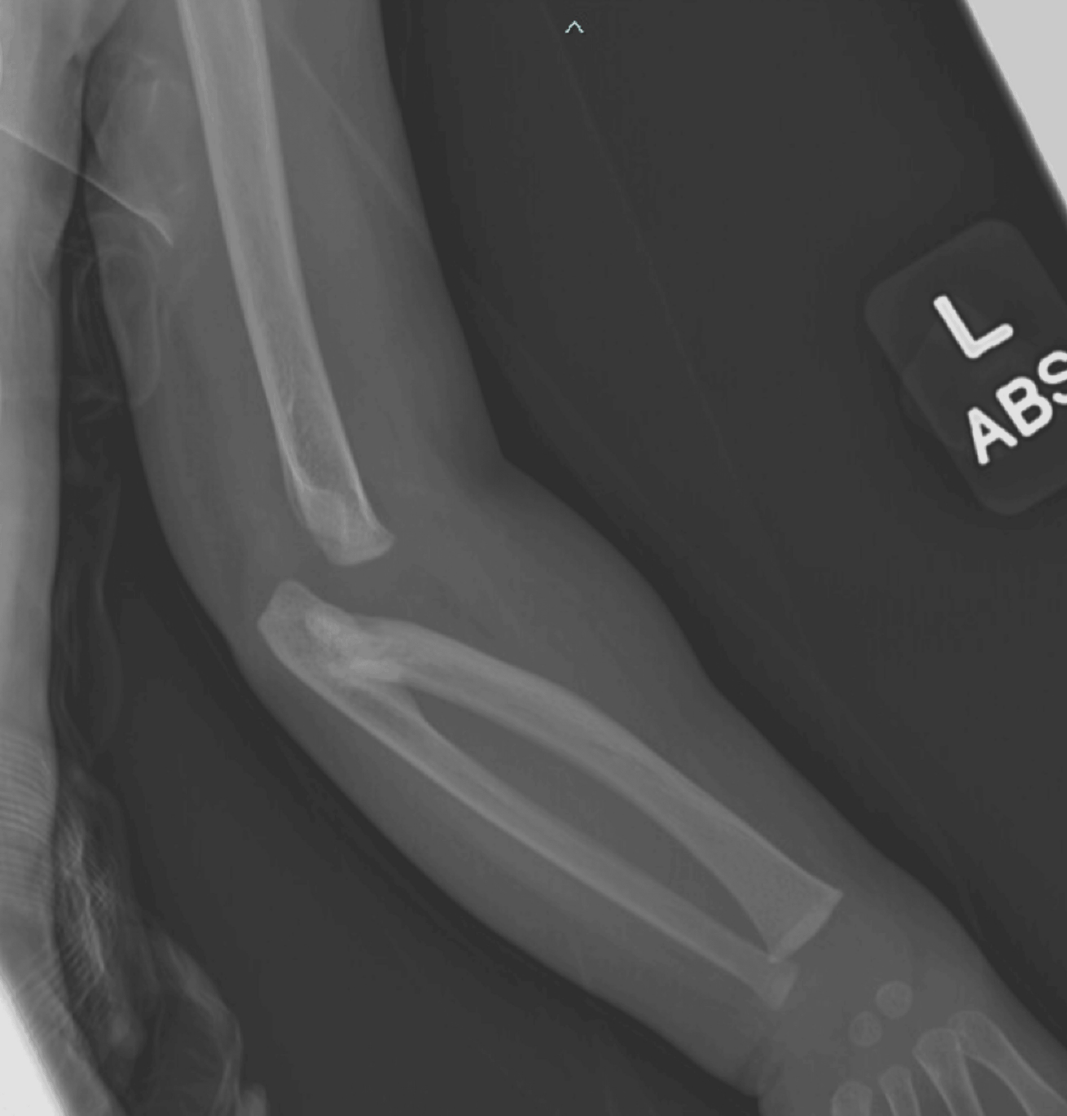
Left-forearm X-ray The distal humerus is dislocated from the radius and ulna. The radial head and olecranon appear to be malformed.
